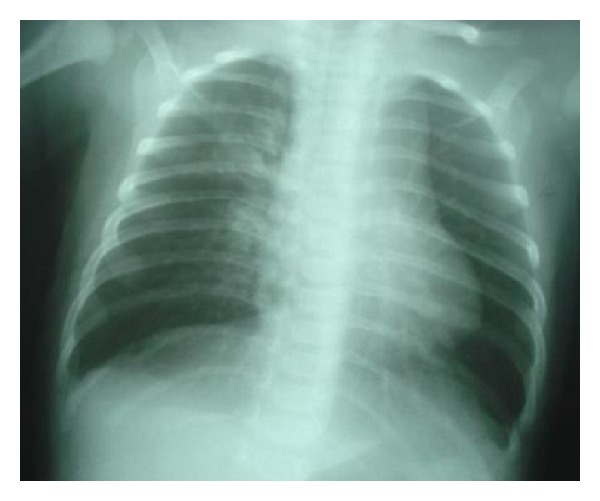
Control chest X-ray: normal.
